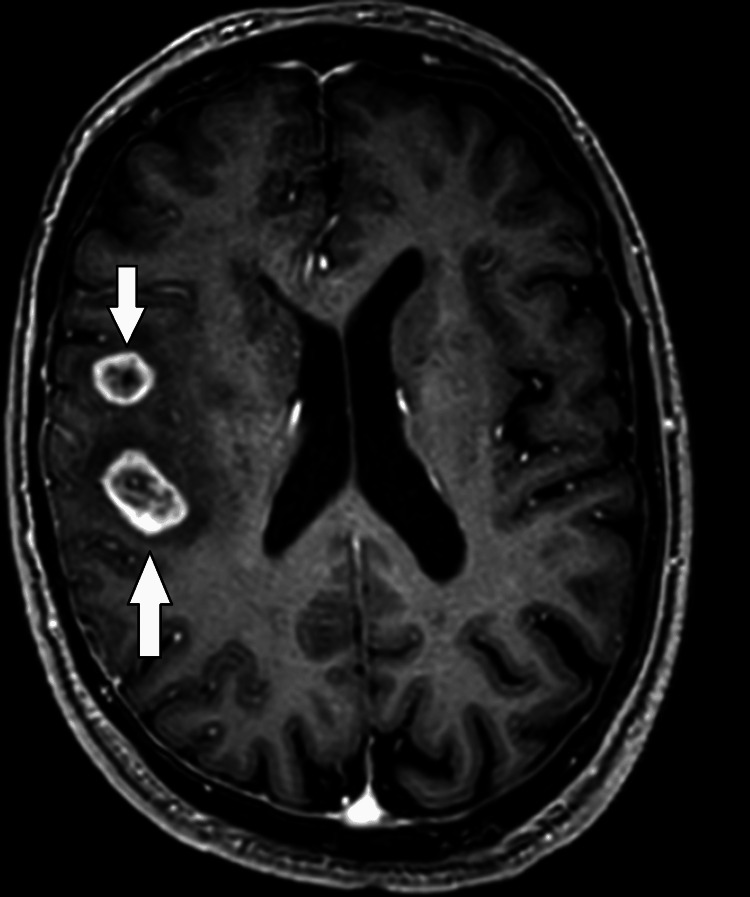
MRI of the head: T1-weighted sequence Peripheral discrete ring-enhancing lesions with central hypointensity were noted, raising suspicions of metastases MRI: magnetic resonance imaging
